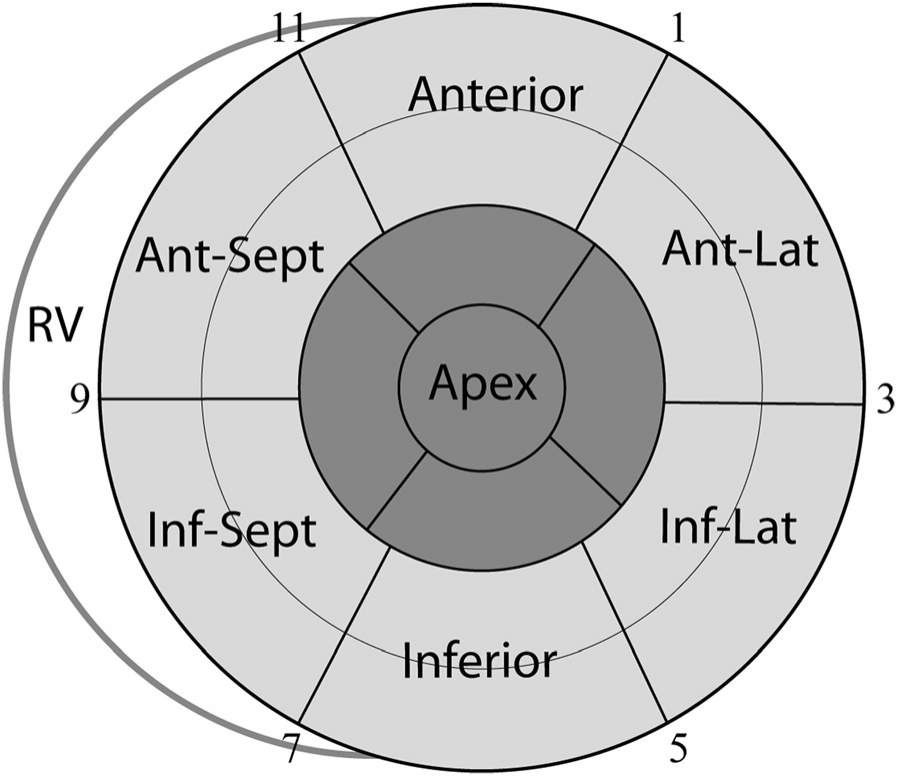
Left ventricular myocardial segmentation. The LV 17-segment model used for SPECT interpretation. Basal and mid-LV segments depicted in light gray are considered equal for LV lead placement. Apical segments and apex are depicted in dark gray. Numbers are in accordance with the clockwise method used for the fluoroscopic venogram in the left anterior oblique projection. The antero-lateral, infero-lateral and infero-septal segments correspond to the echocardiographic lateral, posterior and septal segments, respectively. Ant-Lat, antero-lateral; Inf-Lat, infero-lateral; Inf-Sept, infero-septal; RV, right ventricle.
